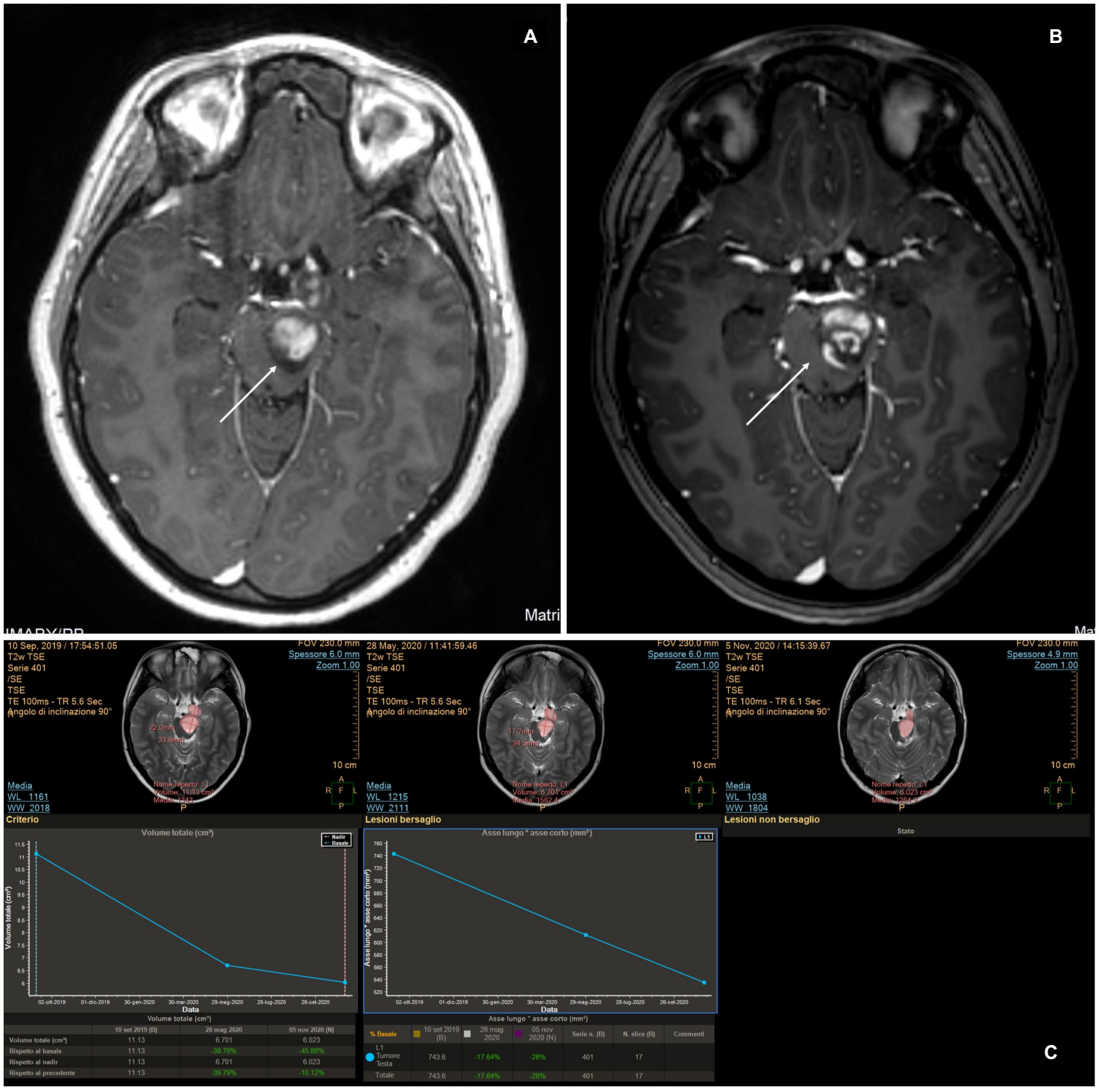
(A) Pre-operative magnetic resonance imaging (MRI). (B) MRI at 48 h after magnetic resonance-guided laser interstitial thermal therapy. A change in the post-contrast enhancement is made evident, compared to the pre-operative scan in panel (A). A lack of enhancement in the central portion of the tumor where the thermal effect induced coagulation and necrosis can be observed. (C) MRI examined with the MITT Intellispace Portal. At 12 months after surgery, the tumor was found to have reduced by 46% in volume compared to pre-operative imaging and by 11% compared to the scan 6 months after surgery.
